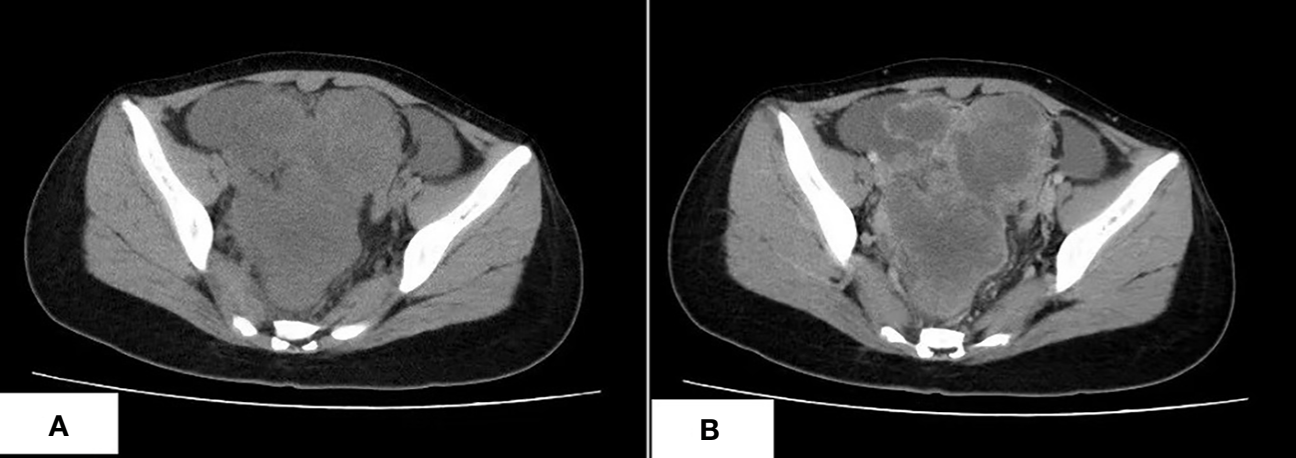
The CT scan of CDS. (A) An irregular lobulated mass in pelvic cavity, which has unclear margin with peripheral structure, is shown. (B) The tumor shows heterogeneous enhancement in the contrast-enhanced CT scan.
